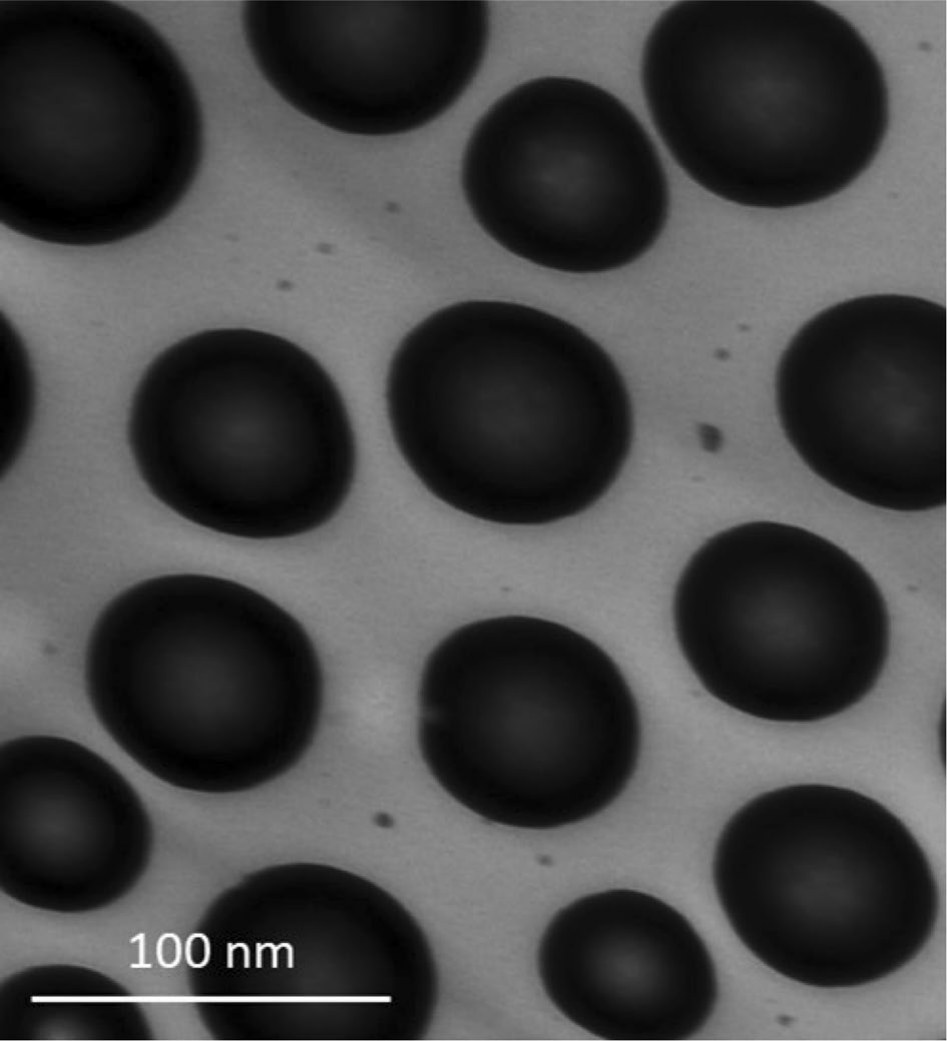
TEM: morphological characterization of CT-CTO-NE4. Image of scale bar 100 nm at 64,000 magnifications.
